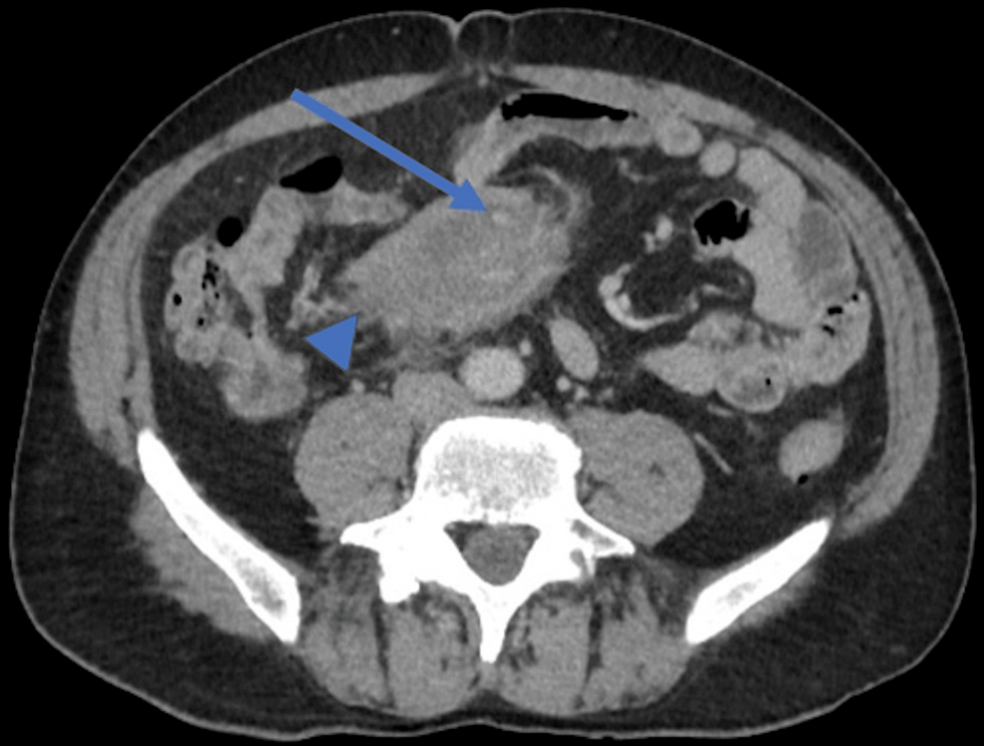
Initial contrast-enhanced computed tomography (CECT) CECT soft mesenteric root mass (arrowhead) displaying a small amount of preserved fat around a mesenteric vessel called the halo sign (arrow).
